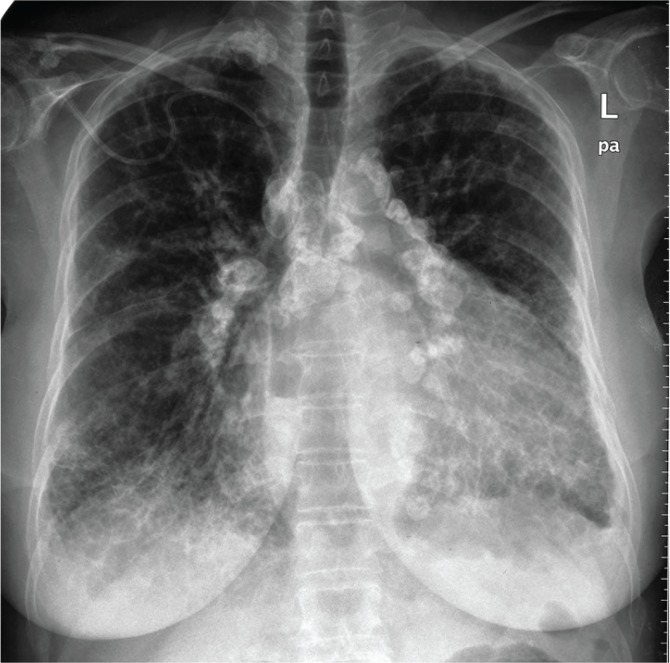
Interestingly, in addition to all the above mentioned typical features of SSc, the patient also had a palpable, hard, supraclavicular lymph node from the beginning of her illness. An extensive work up was performed to rule out malignancy. Chest CT revealed extensive mediastinal lymphadenopathy. Notably, lymph nodes were calcified in an “eggshell” pattern. Supraclavicular lymph node biopsy ruled out malignancy; moreover, no evidence of granulomatous disease was found.
